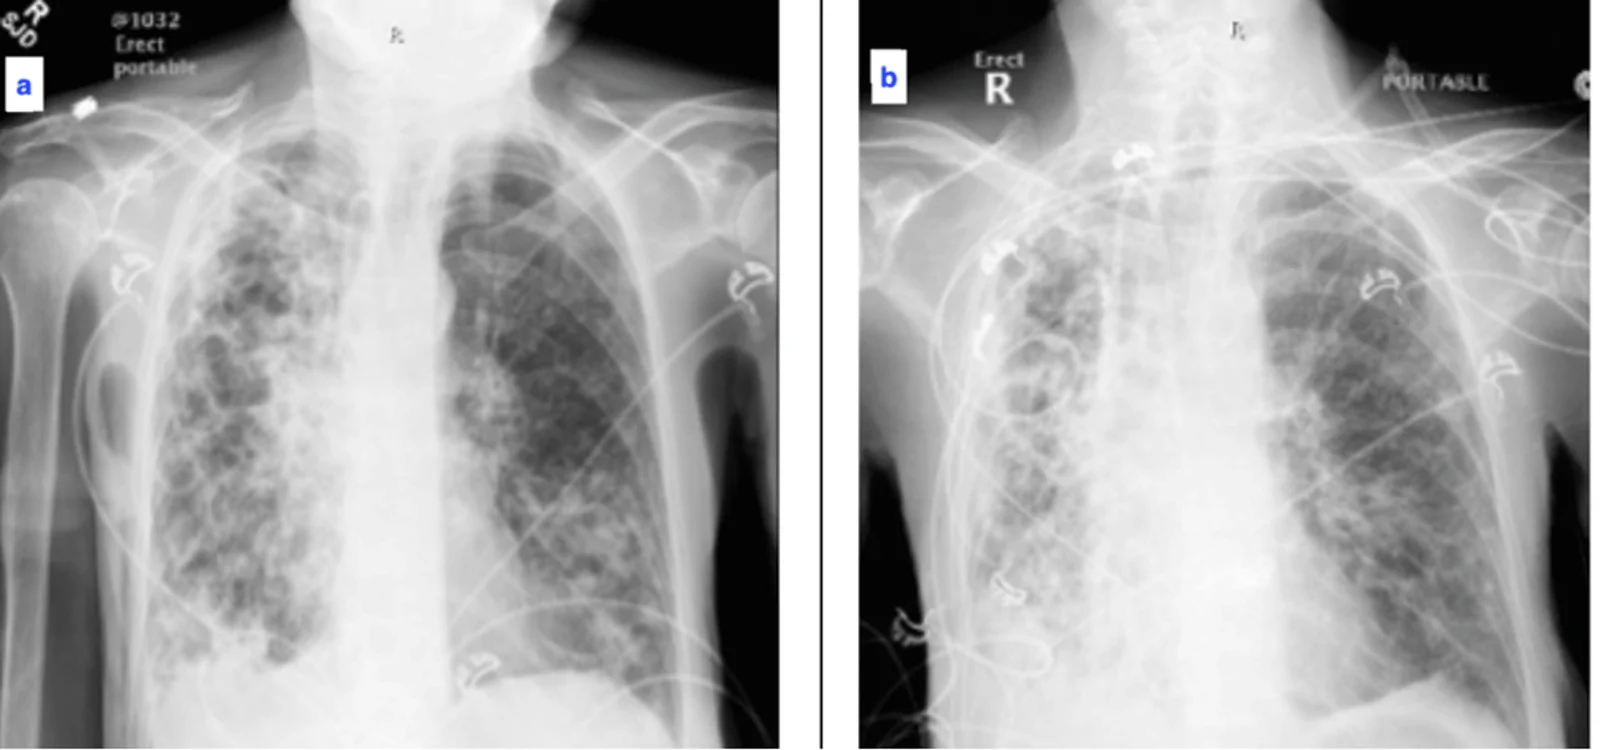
Chest radiographs demonstrating interval progression of pulmonary disease. (a) Initial radiograph demonstrating bilateral pulmonary infiltrates, more prominent in the right lung. (b) Follow-up chest radiograph obtained one week after admission demonstrating worsening aeration and increasing nodular infiltrates involving the left lingula and left lower lobe.
